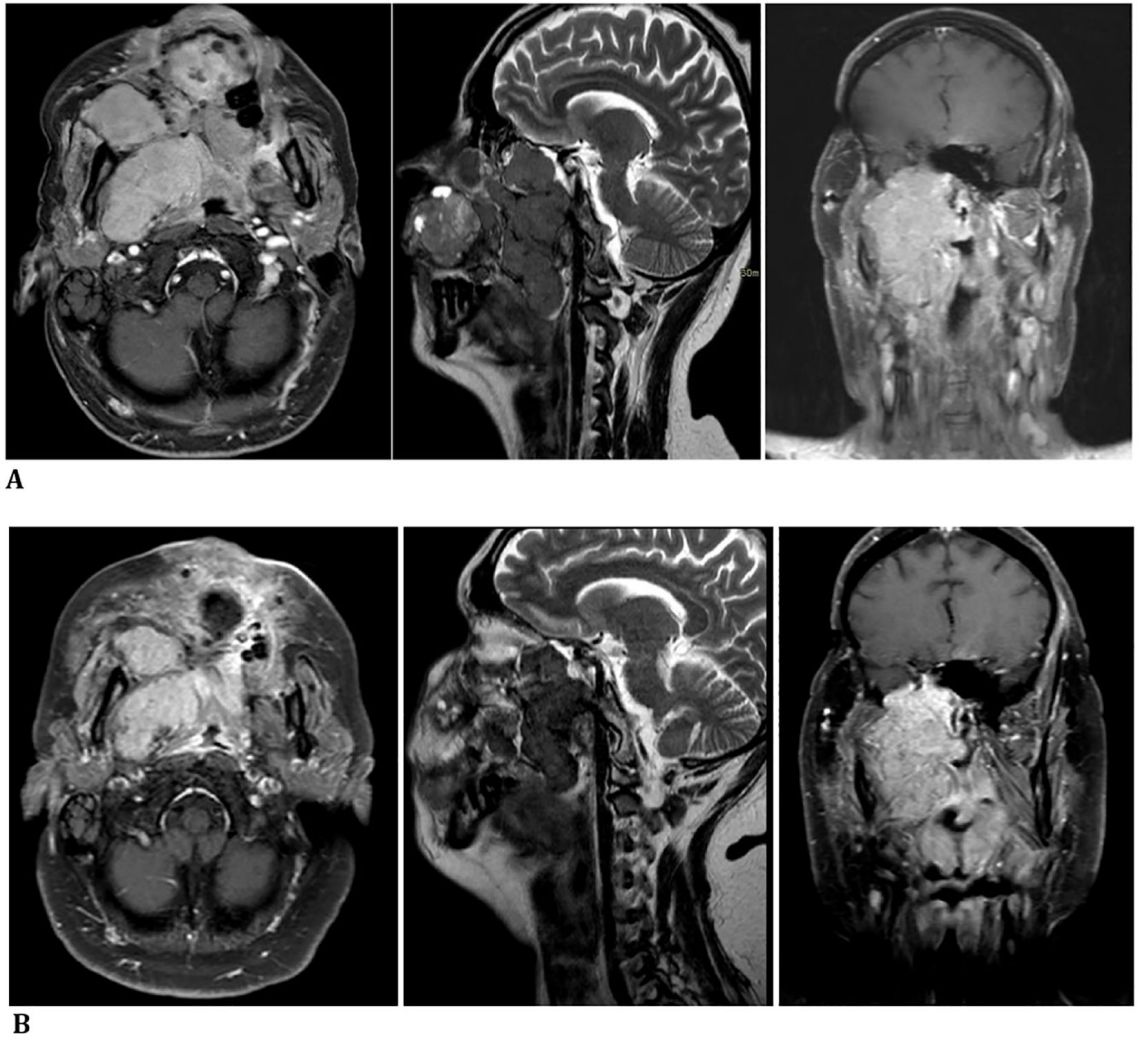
(A) October 2023—progression of disease confirmed by a facial massif MRI before carbon ion therapy. (B) December 2023—radiological reduction in tumor size shown in a facial massif MRI after carbon ion therapy.
